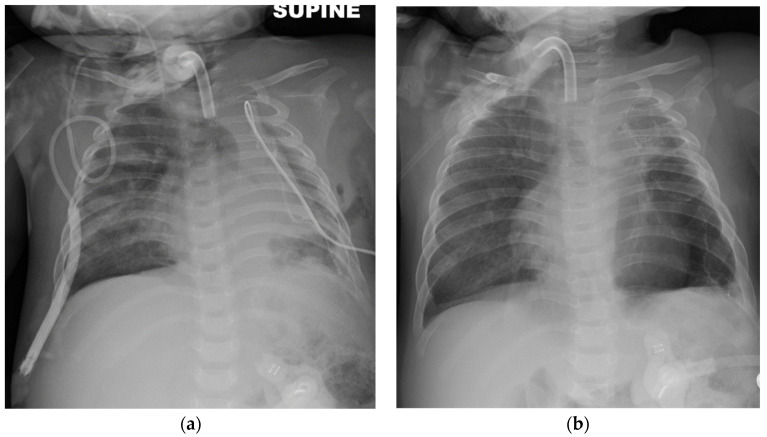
Improvement in right-lung expansion following surgery. (a) In the immediate postoperative chest X-ray and (b) chest X-ray close to discharge.
